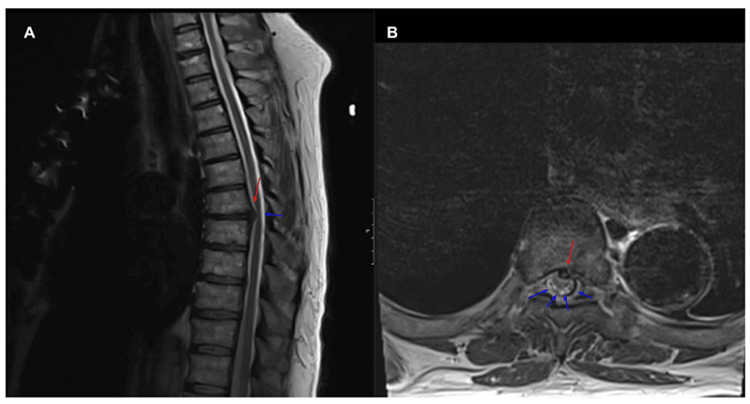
Thoracic spinal cord MRI Sagittal (A) and axial (B) T2-sequences of a thoracic spinal cord magnetic resonance imaging (MRI) without contrast demonstrating a disc herniation at the T7-8 interspace abutting the spinal cord eccentrically on the left (red arrow). There is space within the thecal sac seen dorsally (blue arrows).
